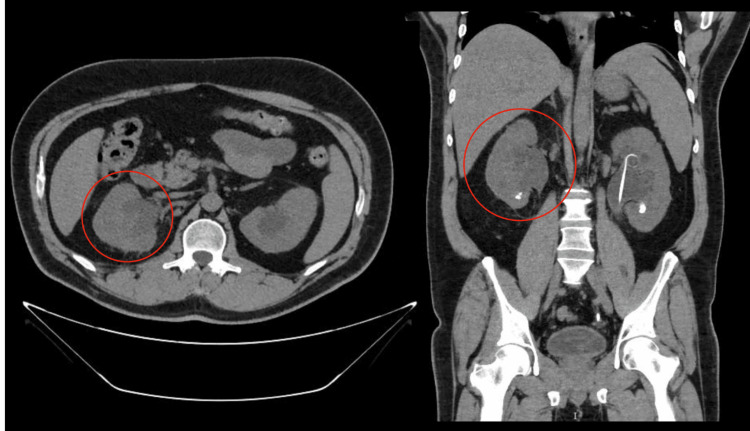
Axial and coronal views of a repeat CT of the abdomen without contrast done three months later of the episode showing near-complete resolution of the previously seen right perinephric hematoma (red circles), along with interval removal of the right double-J stent, and bilateral renal pelvic dilation, similar to prior examinations CT: computed tomography
